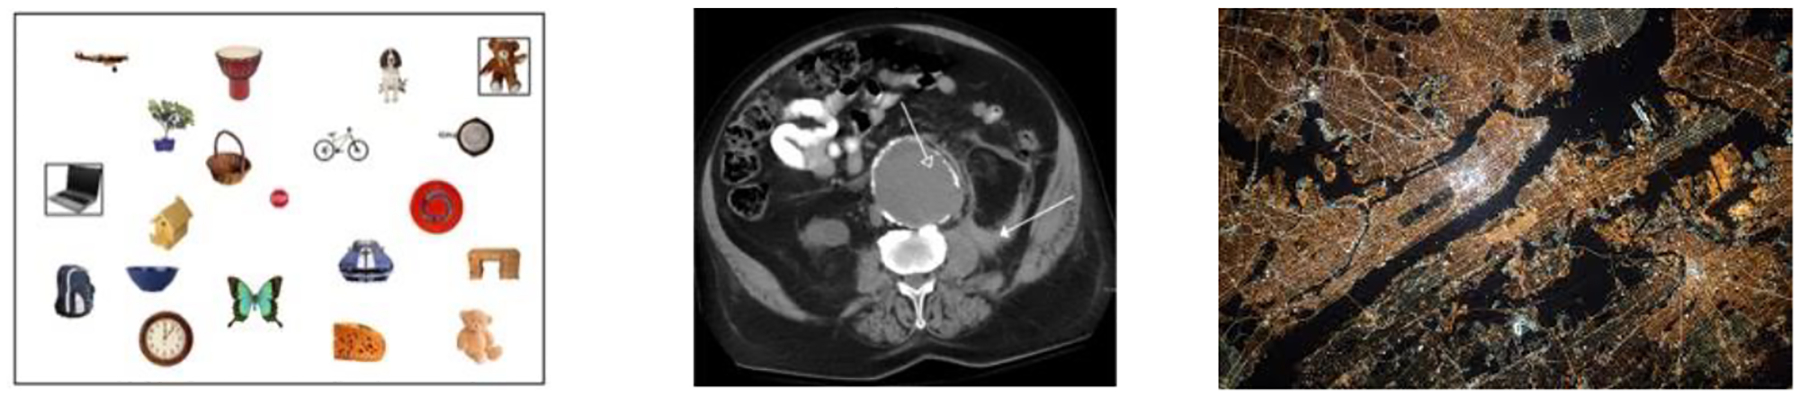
Examples of a typical laboratory visual search array (left; from [21]), an abdominal CT scan (middle; from James Heilman, MD, Wikimedia Commons), and an aerial map (https://pxhere.com/en/photo/919491).
